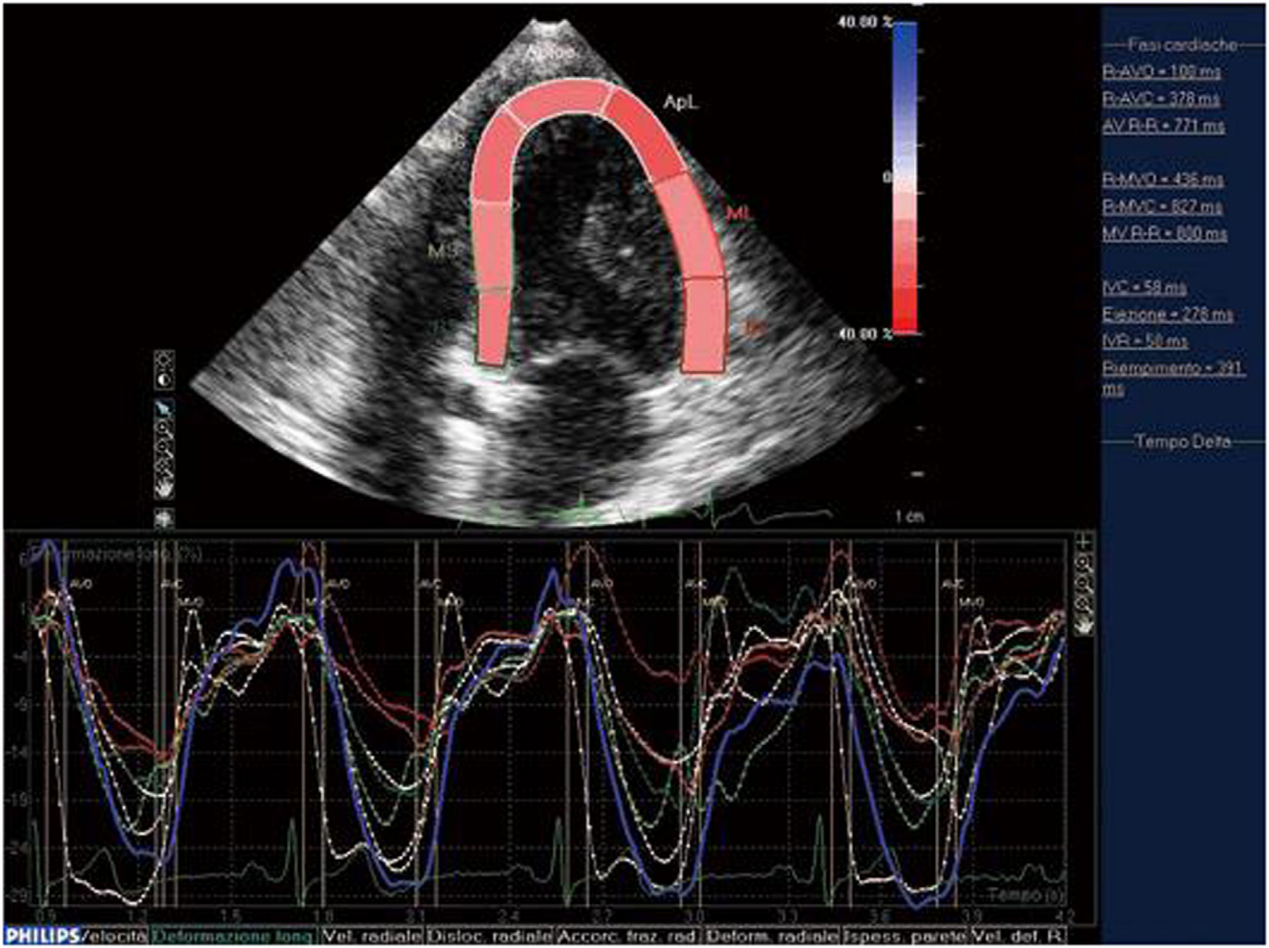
STE: systolic myocardial deformation after electromechanical activation. LV: longitudinal strain from the apical four-chamber view: time–strain curves show a negative end-systolic strain representing myocardial shortening during systole.
